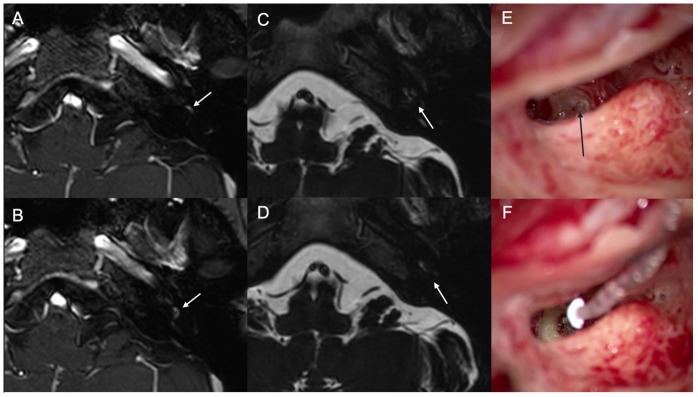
Case #2 (A–F). Preoperative T1-weighted MR with gadolinium enhancement shows the enhancing mass in the basal turn of the cochlea (A) and vestibule (B) (white arrows). T2-weighted MR image shows a filling defect in the basal turn of the cochlea (C) and vestibule (D) (white arrows). Through the round window, a soft tissue mass is shown (black arrow) (E). After removal of the mass, the electrode of the cochlear implant is introduced and fully inserted (F).
